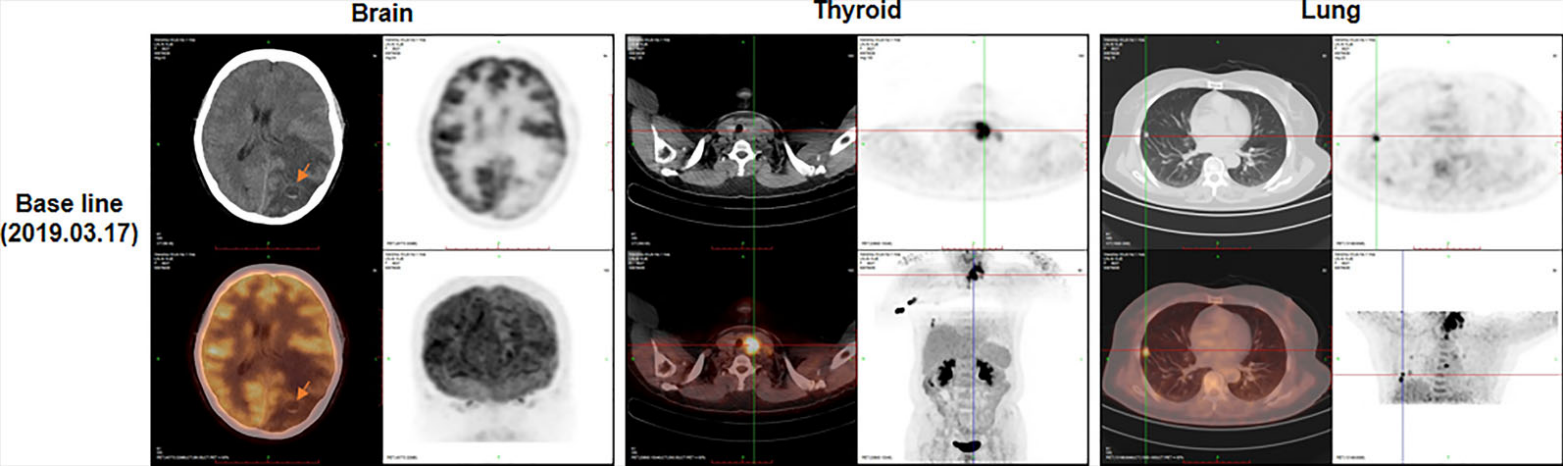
The positron emission tomography-computed tomography (PET-CT) at baseline (before surgery). PET-CT showed masses in brain (arrow), thyroid and lung.
